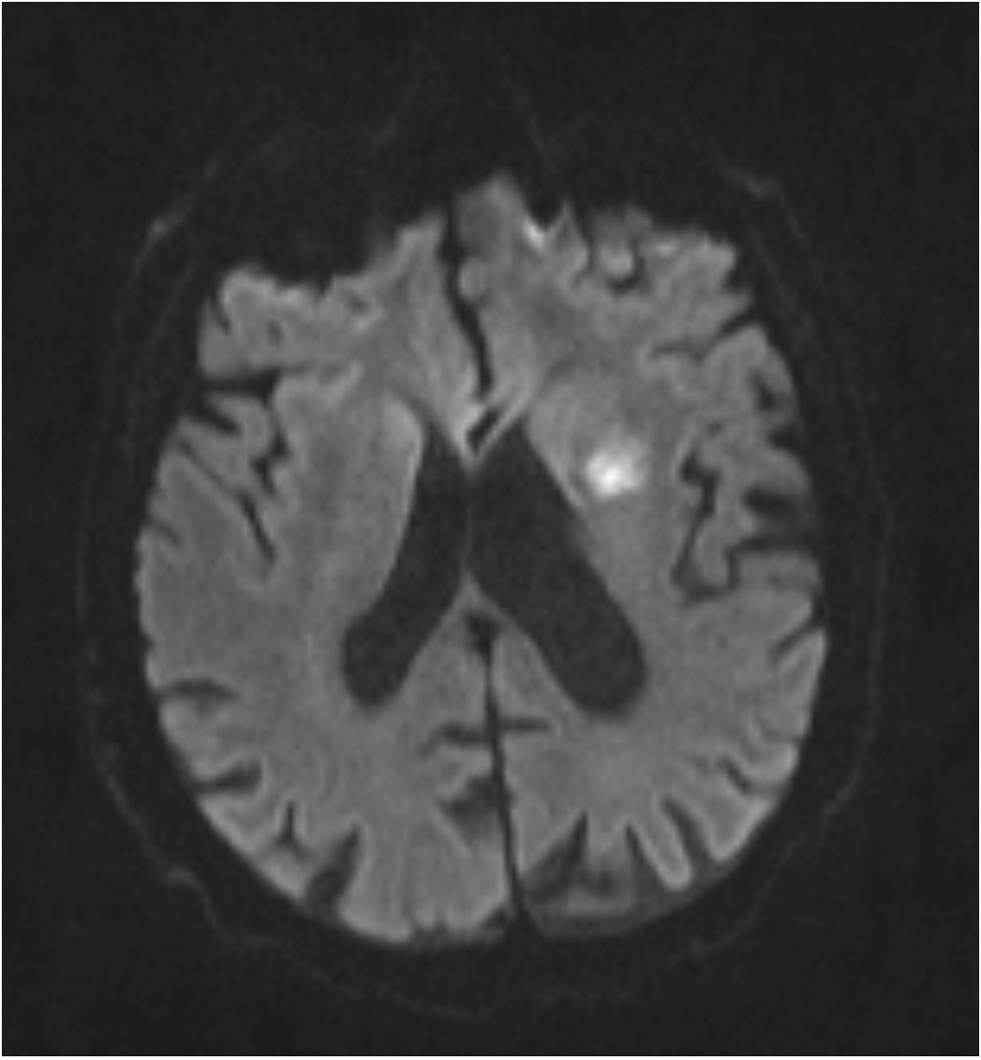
MRI DWI sequence of Perforator Infarct.
